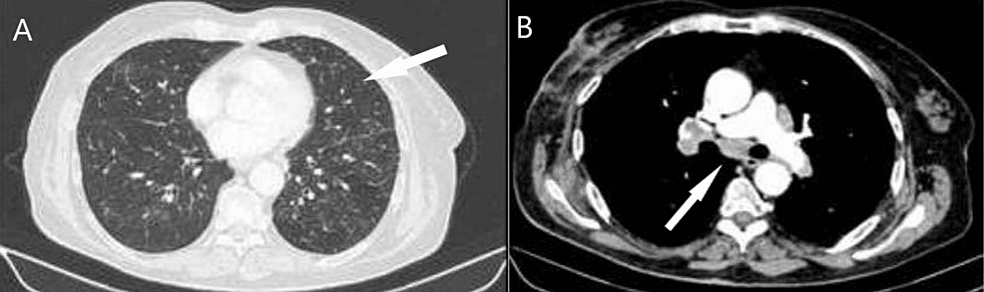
Chest CT. A: Chest CT revealed bilateral micronodules (arrow); B: Chest CT revealed enlarged mediastinal lymph nodes (arrow). CT, computed tomography
